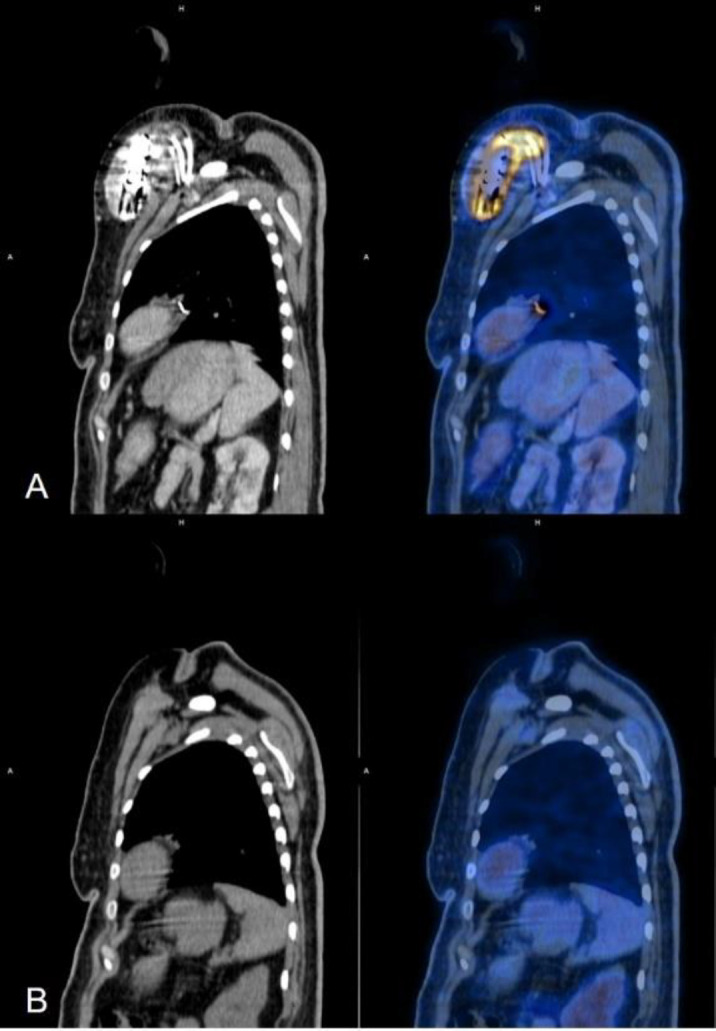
Saggital section CT and fused FDG PET CT images (left to right); (A) showing abnormal increased FDG uptake (SUV_Max_: 8.6) in hypodense collection around the pacing box with extension of the FDG uptake along the adjacent leads. (B) Follow-up FDG PET CT done 4 months later (post explantation of device) showing no abnormal increased FDG uptake at post-surgical site
